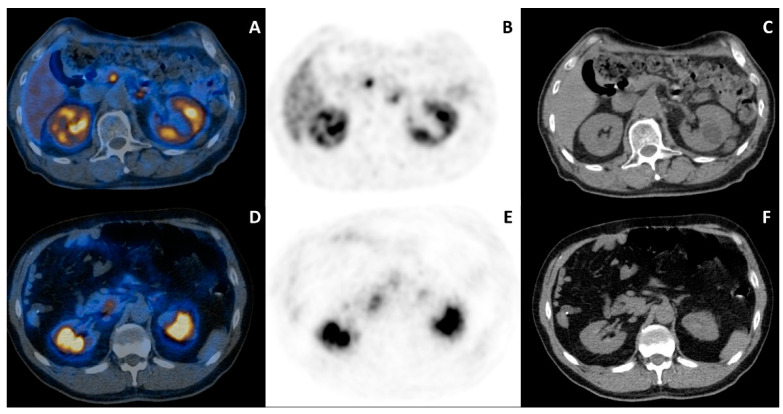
[68Ga]Ga-DOTANOC PET/CT transaxial images (fused PET/CT: (A,D), PET: (B,E), low-dose CT: (C,F)) of two patients showing different patterns of UP uptake. Images of a 76-year-old male pt presenting with a focal UP uptake pattern are shown in (A–C). Note that the intensity of UP uptake is higher than the adjacent normal liver background. On the contrary, a 57-year-old male presented a diffuse UP uptake pattern (D–F). The interpretation of a physiologic uptake is much easier when a diffuse pattern is observed.
